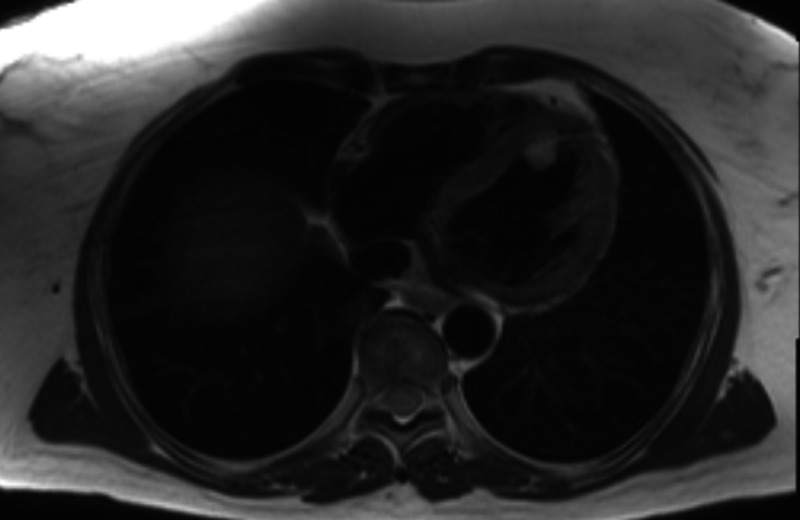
Cardiac MRI transverse view- clearly demonstrated LV apical septal mass
